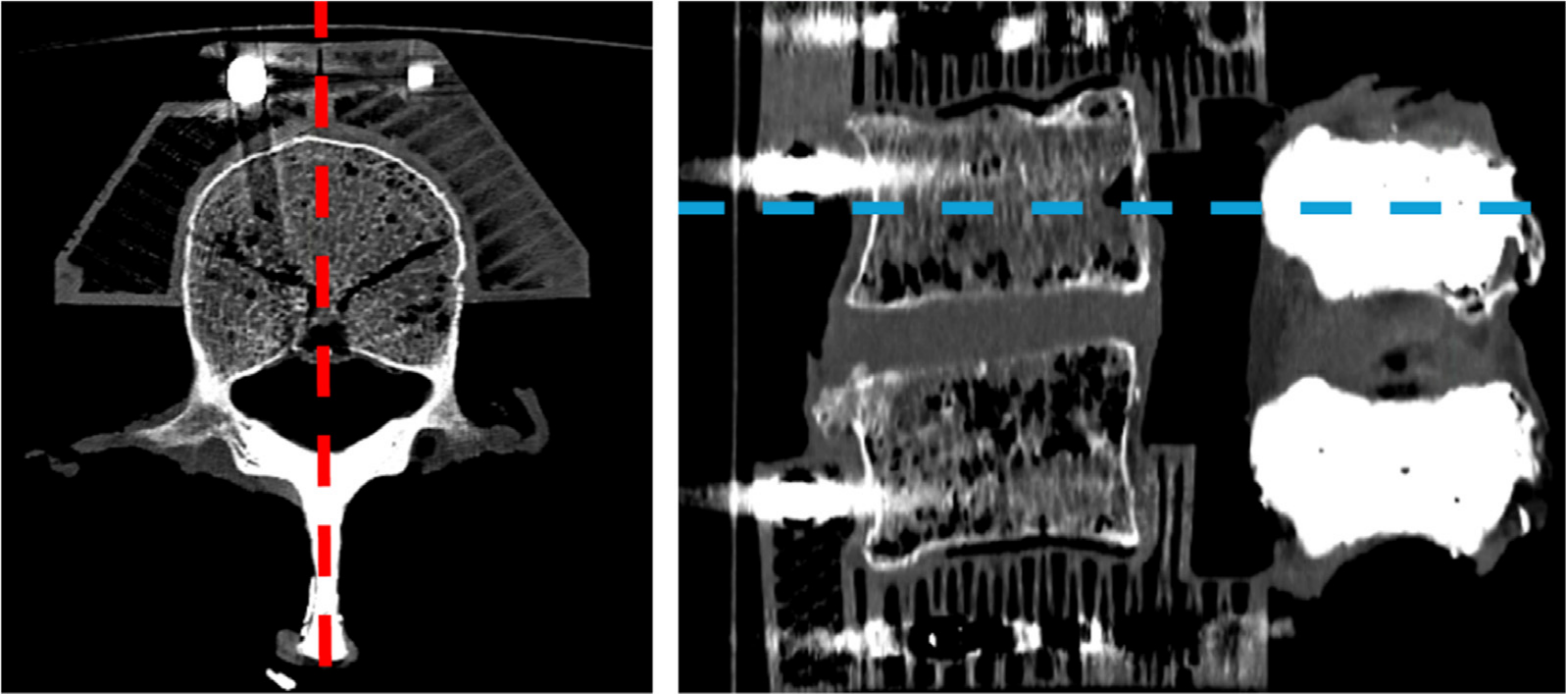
The complete augmentation of the spinous process and parts of the lamina with PMMA was clearly visible in the CT scan.
